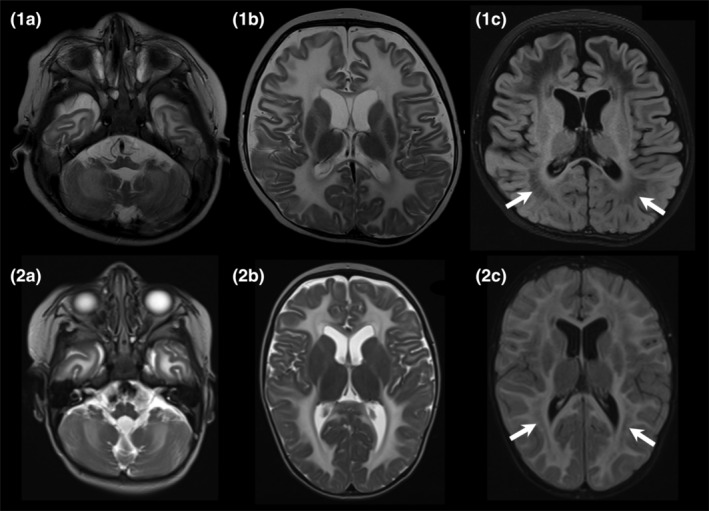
Top row is patient 1 (age 6 months) and bottom row is patient 2 (age 9 months). The images in the two left columns are axial T2‐weighted at 1.5 T showing a diffuse leukoencephalopathy with symmetric high signal throughout the cerebral and cerebellar white matter. There is relative sparing of the internal capsules. The images in the right column are FLAIR sequences showing rarefaction of the white matter (arrows)
